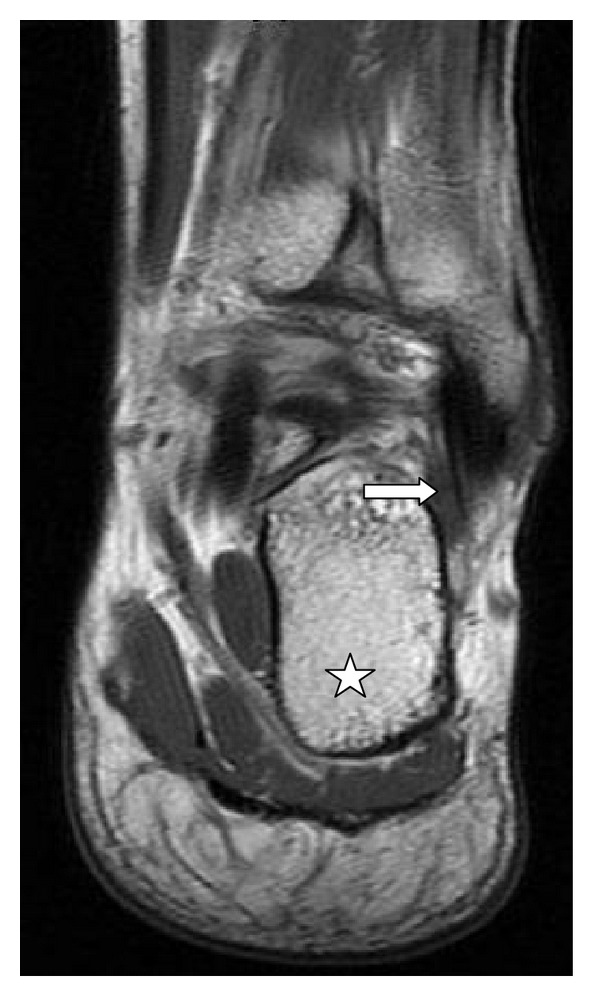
MRI, coronal T1 18/660 of left ankle. Star: calcaneus bone; arrow: fibularis quartus muscle.
